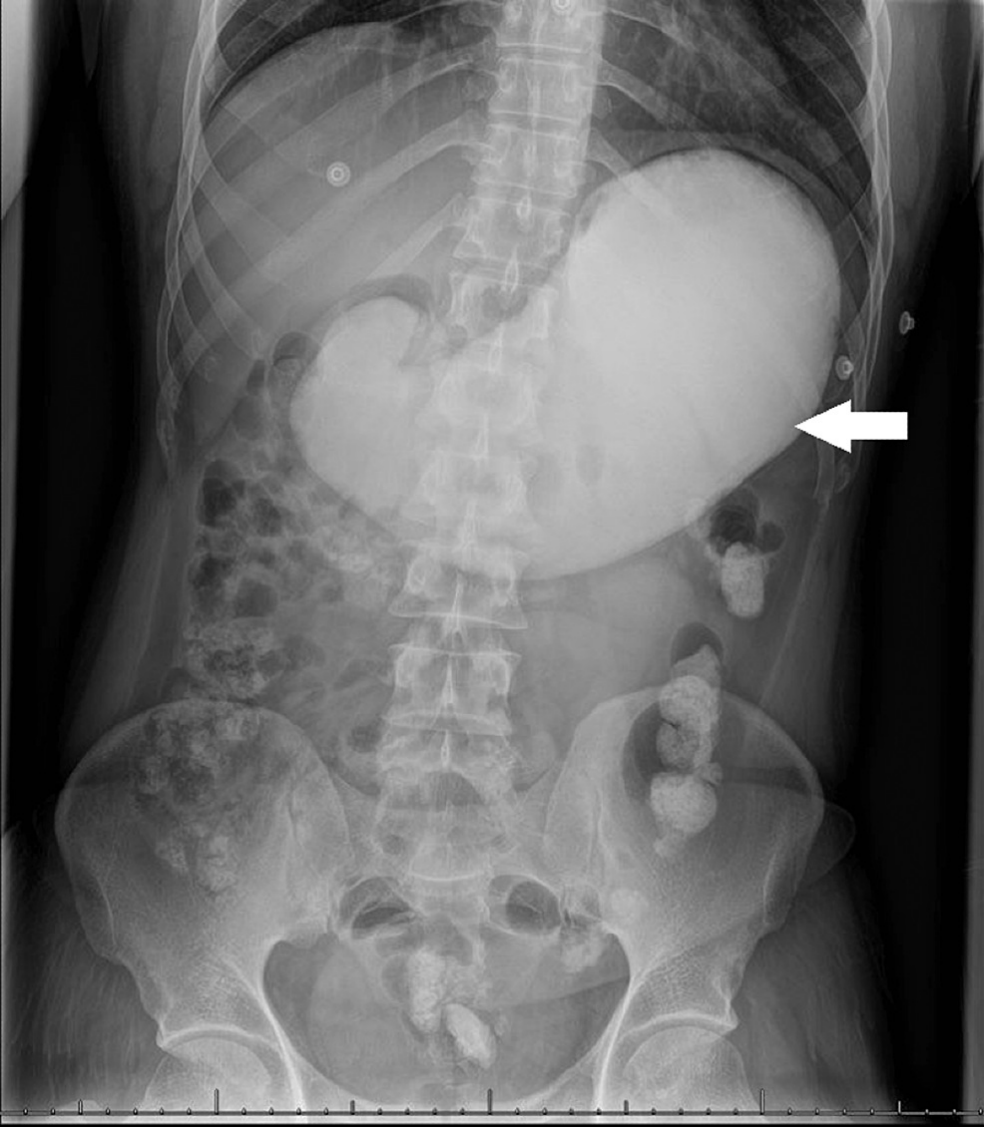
Abdominal X-ray Radiograph of the abdomen (first hospitalization day) showing dilated stomach with radiopaque shadow.
